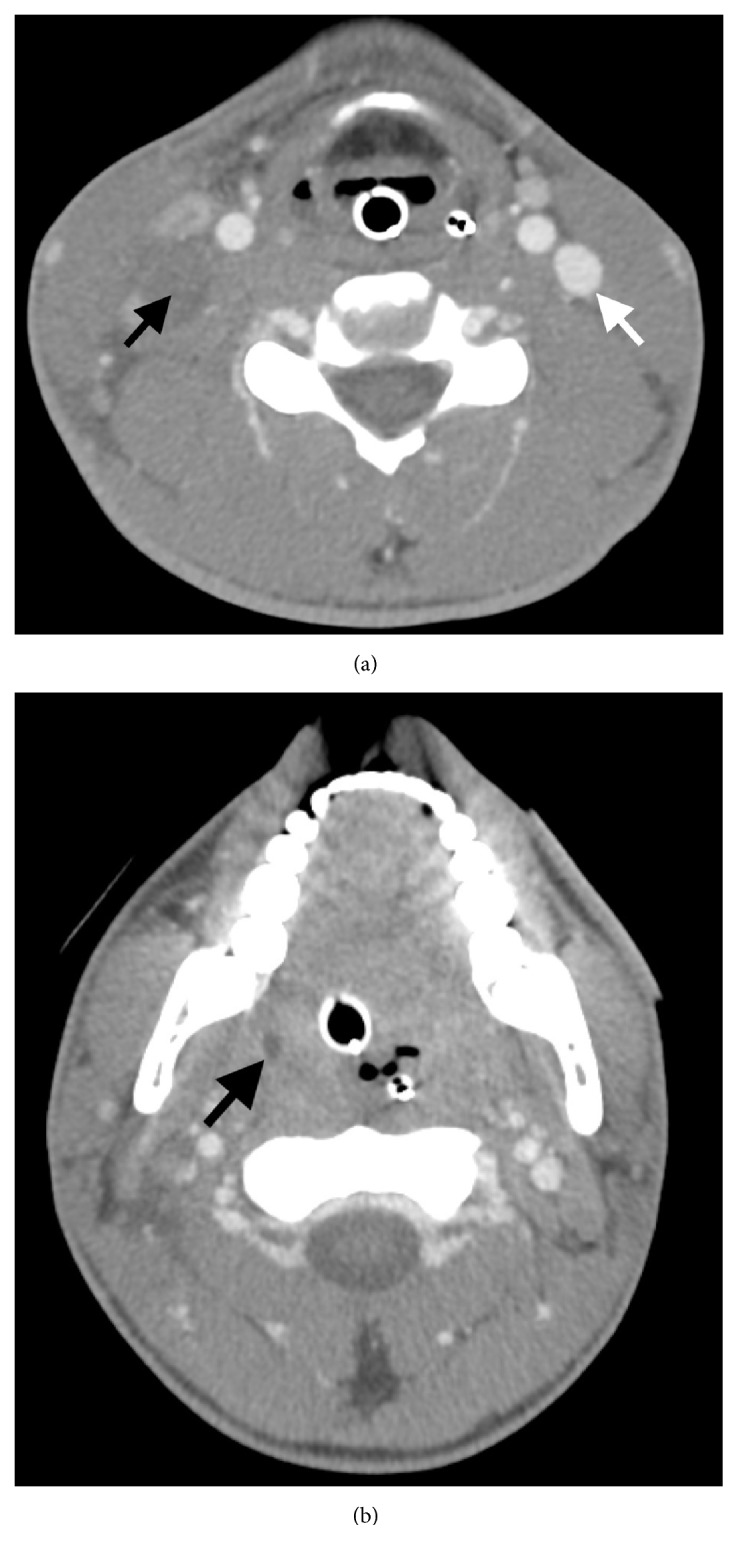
Axial image from CT scan of the neck with intravenous contrast. (a) There is complete occlusion of the right internal jugular vein (black arrow). The left internal jugular vein is patent (white arrow). A small amount of hypodense fluid surrounding the occluded right internal jugular vein reflects inflammatory changes. (b) Peritonsillar hypodensity (black arrow) measured up to 0.6 cm and was presumed to be a small abscess. Endotracheal and enteric tubes are noted.
